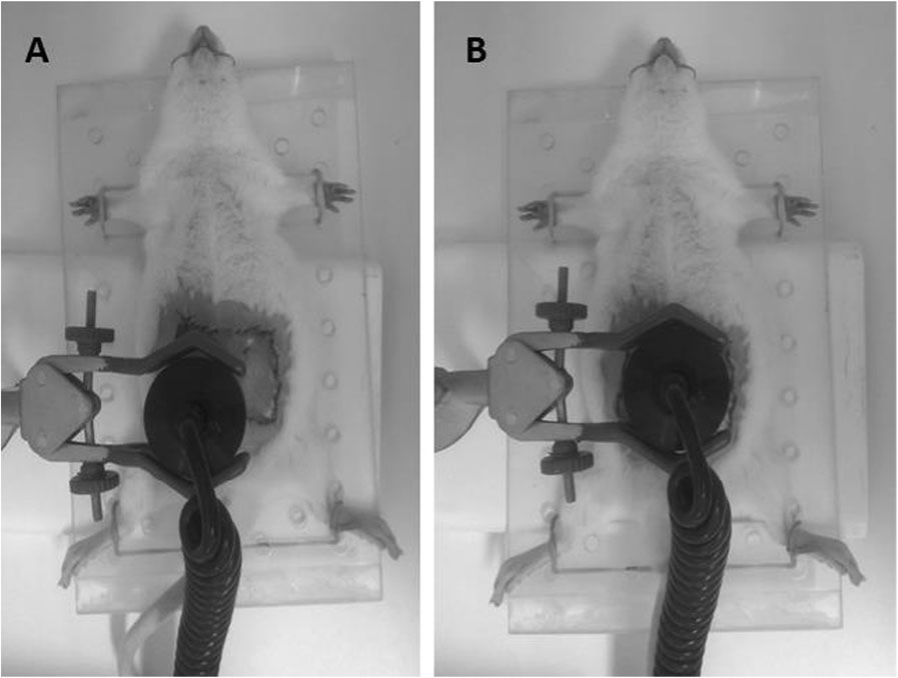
Site of application of the ultrasound transductor. a LIPUS positioned at the flap zone 1 (group 3); b LIPUS positioned at the center of the flap (group 2)
